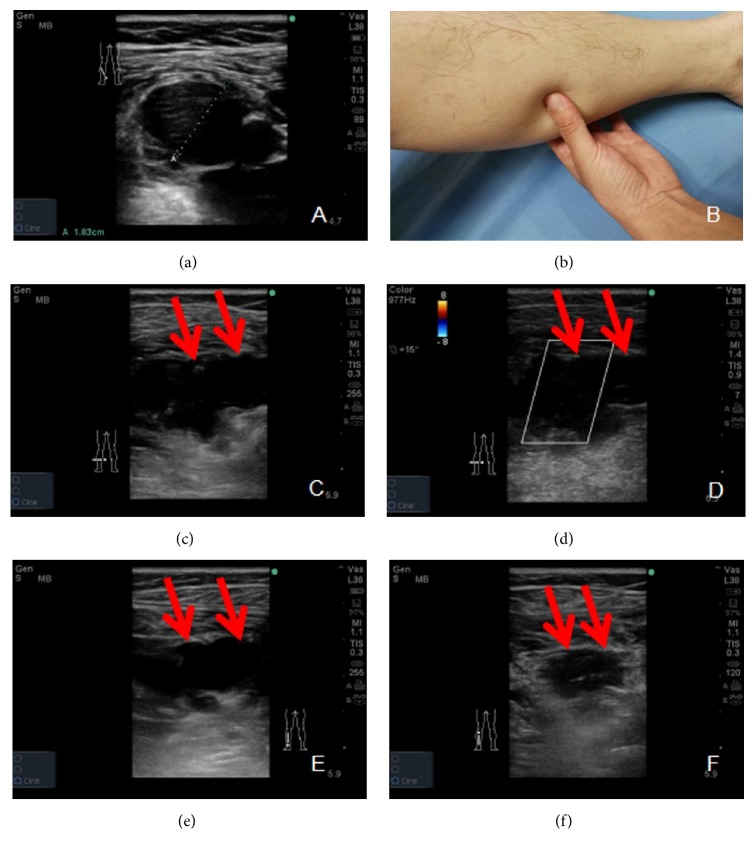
(a–f) A symptomatic deep vein thrombosis (DVT) patient with great preoperative soleal vein (SV) diameter. (a) shows that preoperative SV diameter was 1.83 cm before total hip arthroplasty; (b) shows that, 3 weeks after THA, this patient got back to hospital and presented that he could not walk normally with severe calf pain and physical examination showed homans and neuhof sign were positive; (c-d) the ultrasound screening showed a hypoechoic signals in the transverse view (c) and little blood flow signal can be visualized on color Doppler mode (d) (red arrows); (e) shows, 6 weeks after THA, B-mode shows hypoechoic areas still cannot be compressed (red arrows); (f) shows, 12 weeks after THA, the size of DVT became smaller but still existed (red arrows).
